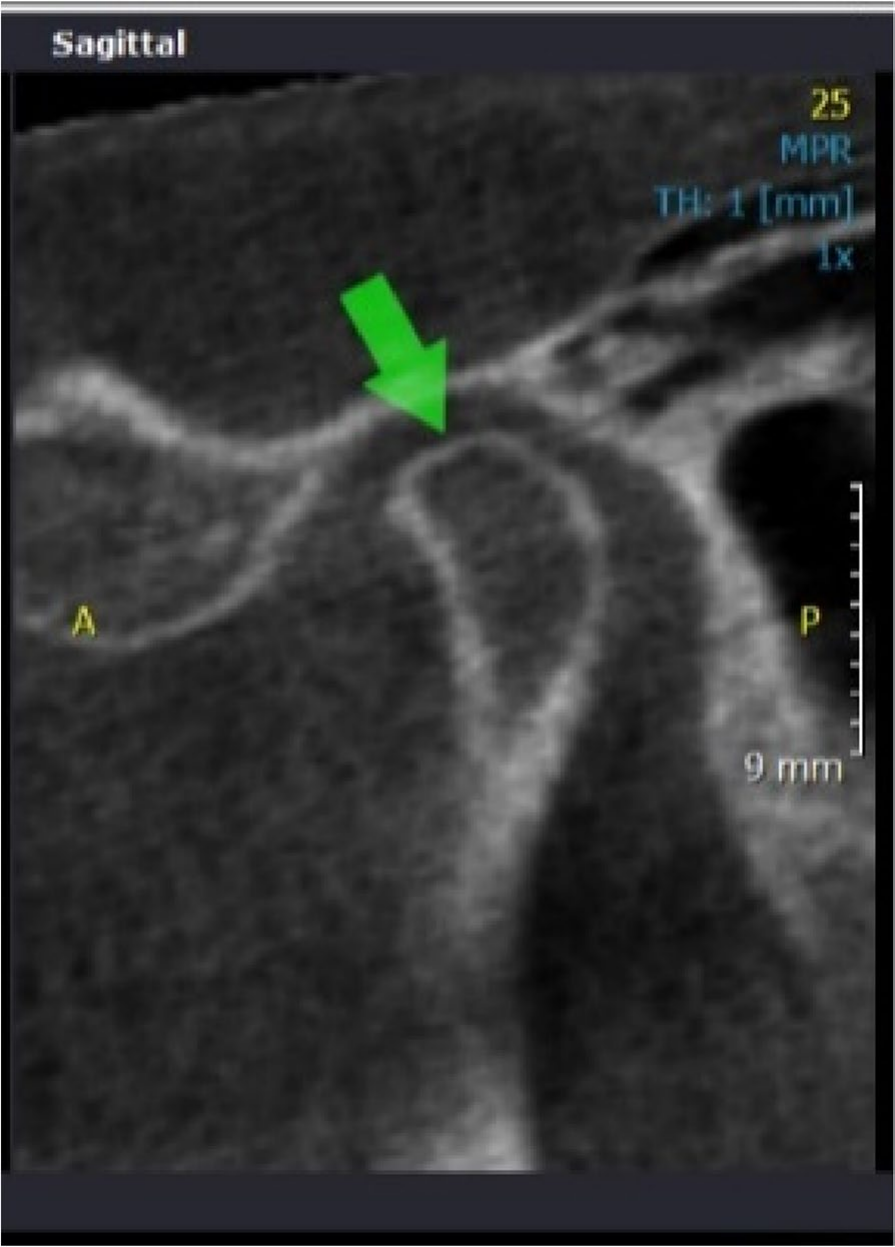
Sagittal CBCT view showing flattening on superior border of left condyle
